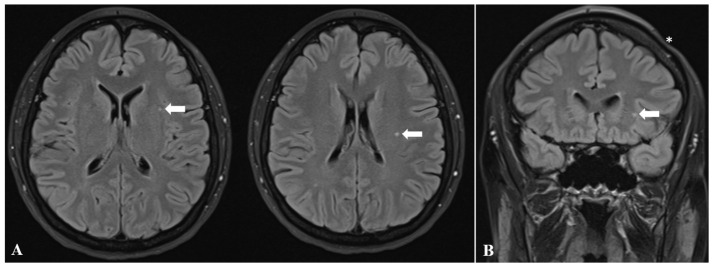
Subcortical white matter lesions in the frontal lobe ipsilateral to the facial atrophy (white arrows), demonstrated on axial FLAIR (A) and coronal FLAIR (B) brain MRI sequences. Cutaneous atrophy is also visible in subfigure B (white asterisk).
